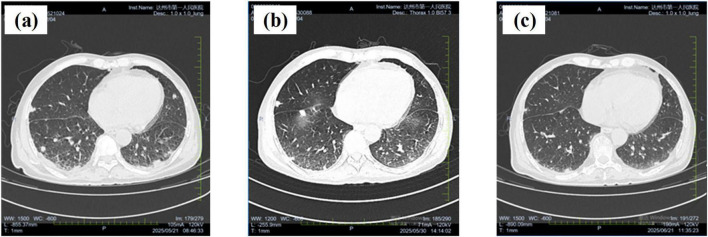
(a) Chest CT on 2025-05-21. (b) Chest CT on 2025-05-30. (c) Chest CT on 2025-06-21.
